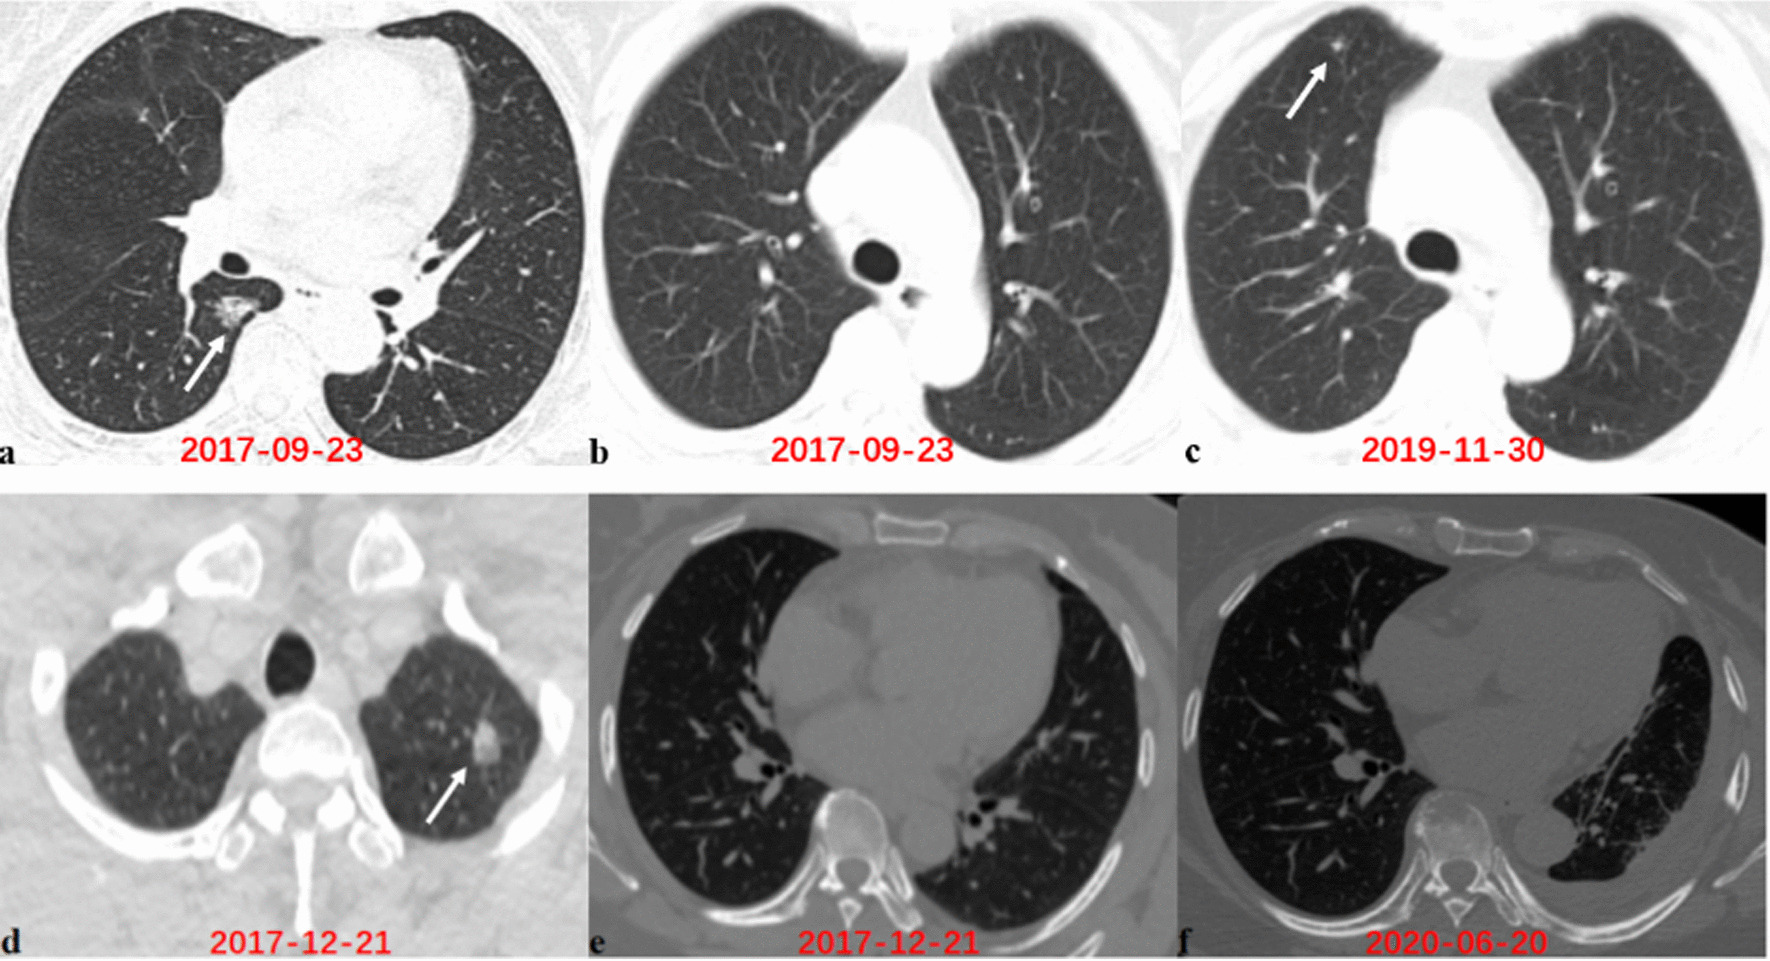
Axial computed tomography images show recurrence during follow-up. First row: A 62 year-old woman with lung adenocarcinoma. a The primary original lesion at the right lower lobe appears as a part-solid nodule with irregular consolidation (white arrow). b–c An emerging nodule diagnosed as metastasis at the right upper lobe (white arrow). Second row: A 55 year-old woman with lung adenocarcinoma (white arrow). d The primary original lesion at the left upper lobe appears as a part-solid nodule with irregular consolidation. e–f Osteolytic destruction of the vertebra and pleural thickening accompanied with pleural effusion both diagnosed as metastasis
